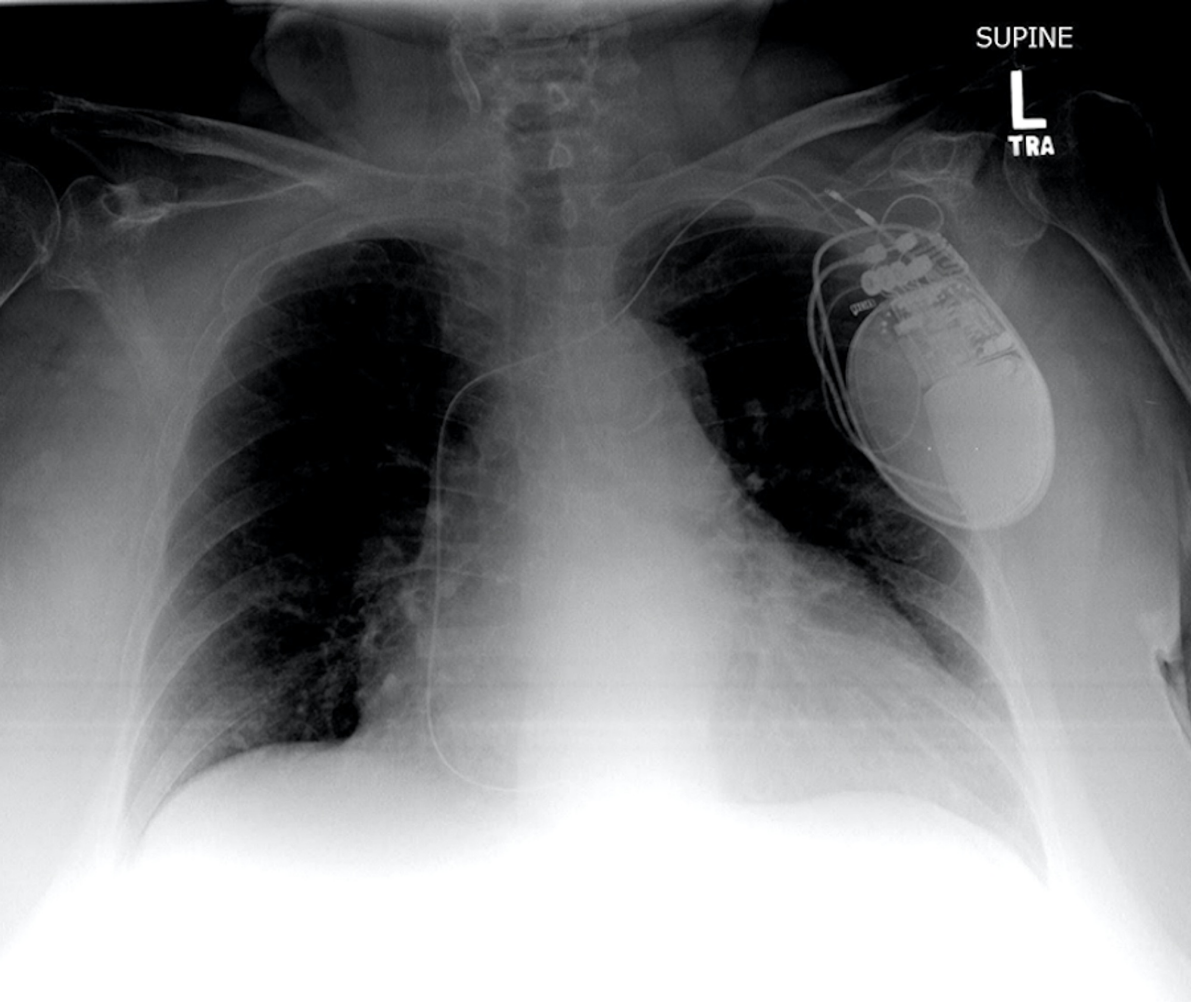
Supine portable radiograph reveals a fractured and coiled lead on the upper portion of the device
